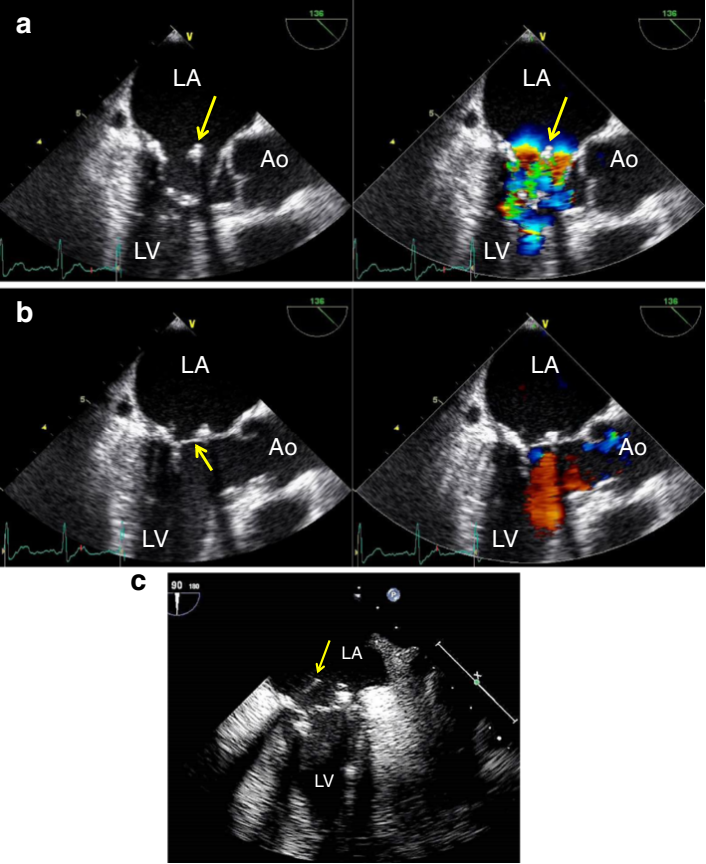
Transoesophageal echocardiography. a. The mitral annuloplasty ring was detached from the mitral annulus at a 136° angle in early diastole (*arrow*). LA = left atrium; LV = left ventricle; Ao = Aorta. b. The mitral valve is re-attached to the annuloplasty ring in early systole (*arrow*); trivial MR is present on colour Doppler echocardiography. c. Transoesophageal, two-chamber echocardiogram showing 15-mm long, highly mobile vegetations (*arrow*)
